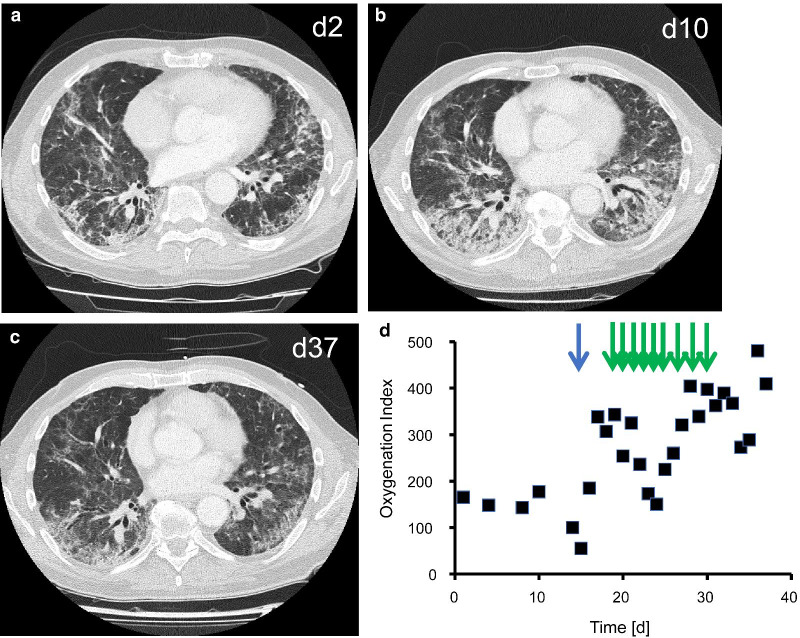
Patient 1. Chest computed tomography scan showing interstitial pneumonia on day 2 (a) and day 10 (b) with mainly axial distribution, subpleural consolidations and no honeycombing. Improvement in the chest computed tomography scan on day 37 (c). There was a close temporal association of the initiation of therapeutic plasma exchange on day 14 (blue arrow), and the nine immunoadsorptions (green arrows from day 19 until day 30) with recovery of oxygenation index (closed squares) (d)
